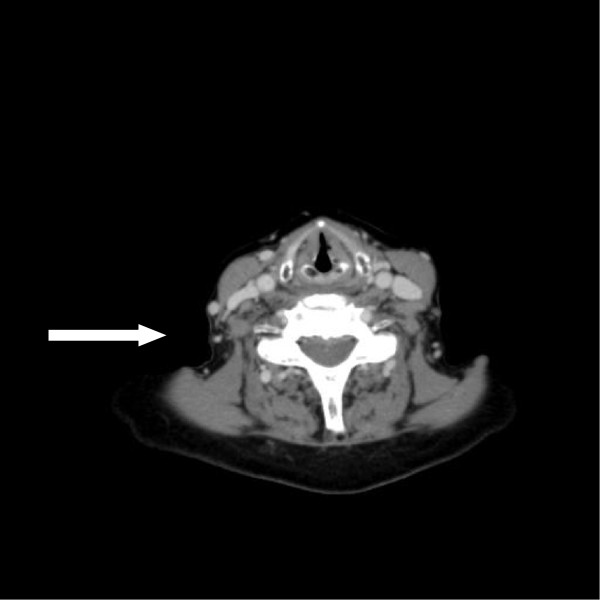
CT scan of the neck after 2 cycles of belinostat (April 2010) showing a complete response.
